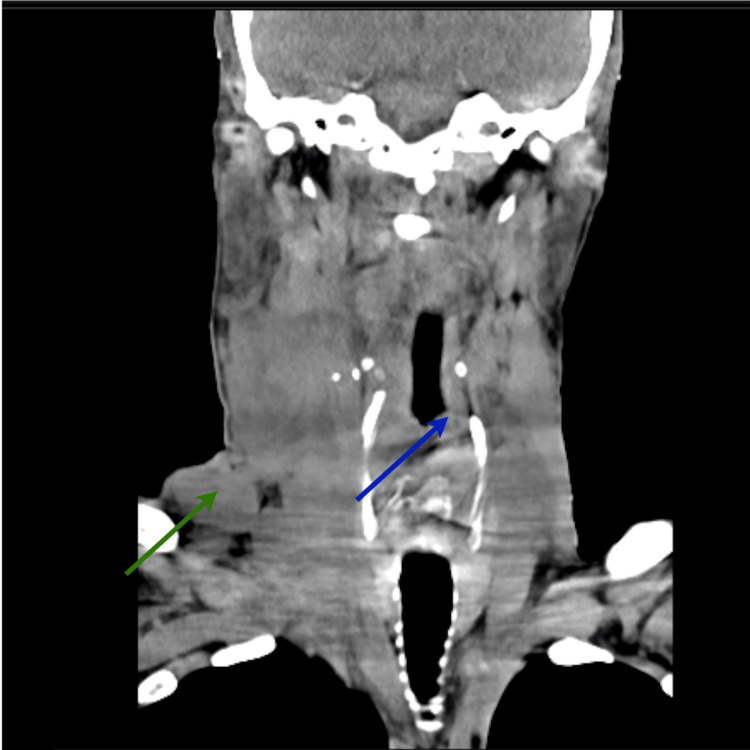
CT scan of the neck, performed in the context of airway obstruction due to a glottic mass, revealed significant narrowing of the laryngeal lumen, invasion of the left laryngeal cartilage, and a conglomerate of cervical lymphadenopathy involving levels II, III, IV, and V, consistent with stage T4N2M1 disease. blue arrow – glottic mass; green arrow – conglomerate of lymph nodes.
